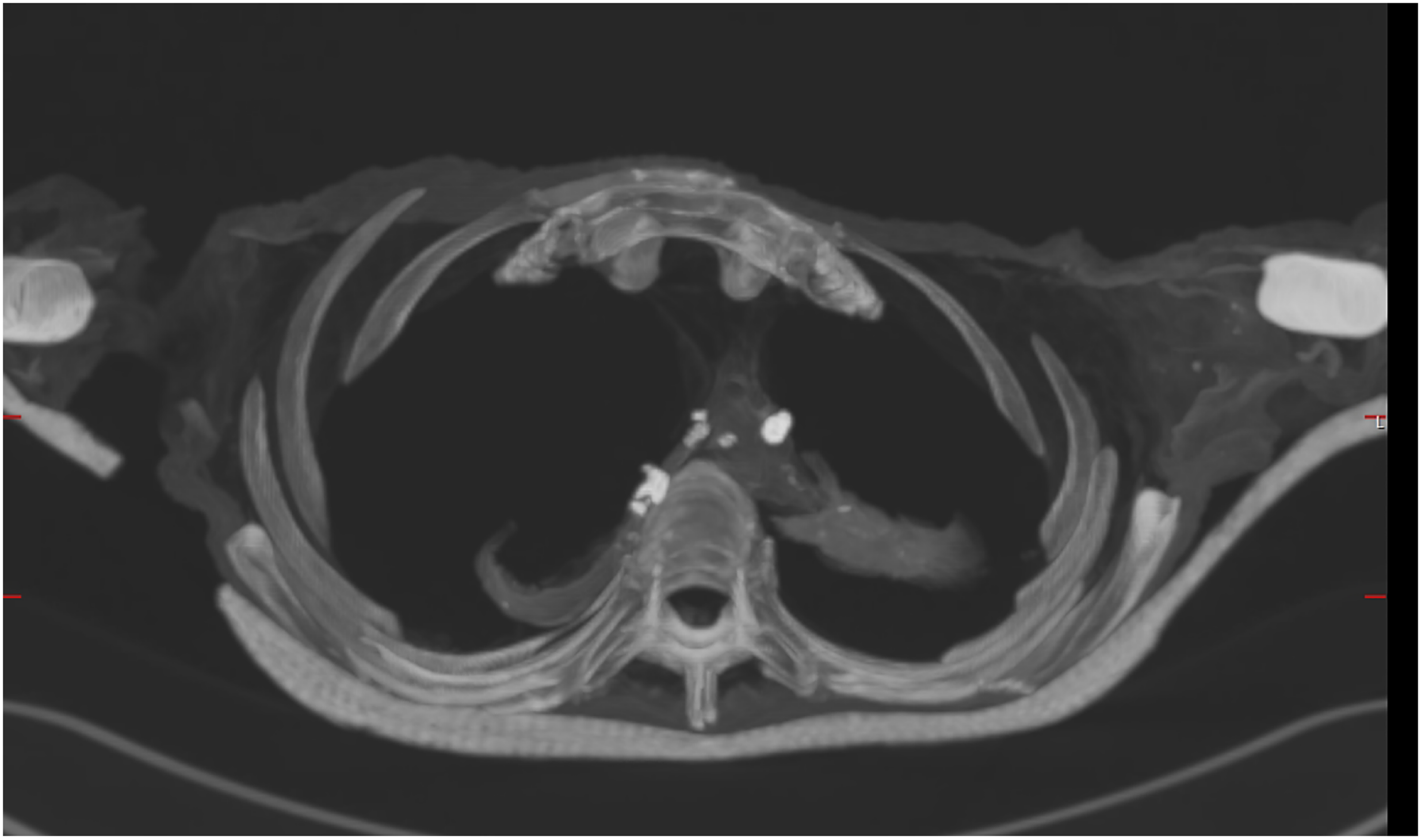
Axial multi-planar MIP reconstruction of a CT data set showing the thorax of individual B. Several calcifications can be seen in the mediastinum, the pulmonary hili and the lungs.
